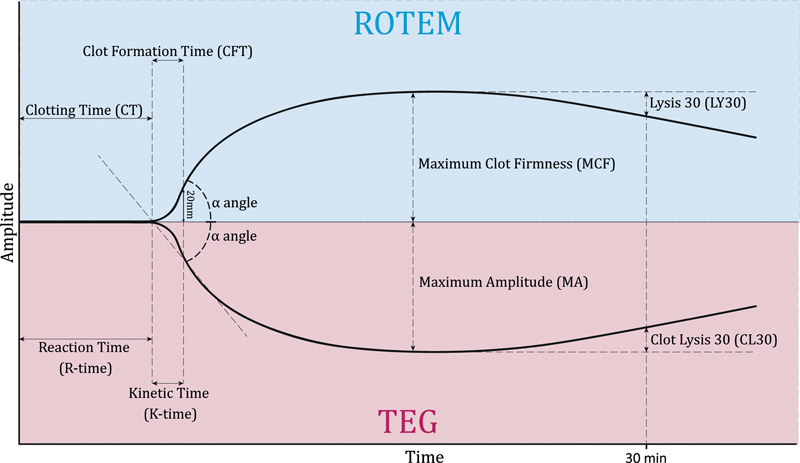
Example of a thromboelastography (TEG) and rotational thromboelastometry (ROTEM) tracing. All parameters reflect on distinct aspects of coagulation. Initiation phase: reaction-time (R-time) and clotting time (CT), the time from start of the assay until an amplitude of 2 mm is reached. Propagation phase: kinetic-time (K-time) and clot formation time (CFT), time from 2-mm amplitude until 20-mm amplitude; a-angle, slope between R-time/CT and K-time/CFT; clot strength, maximal amplitude (MA) and maximal clot firmness (MCF), highest amplitude reached; fibrinolysis, clot lysis 30 (CL30) and lysis 30 (LY30), decrease of amplitude after 30 minutes compared with MA/MCF.
